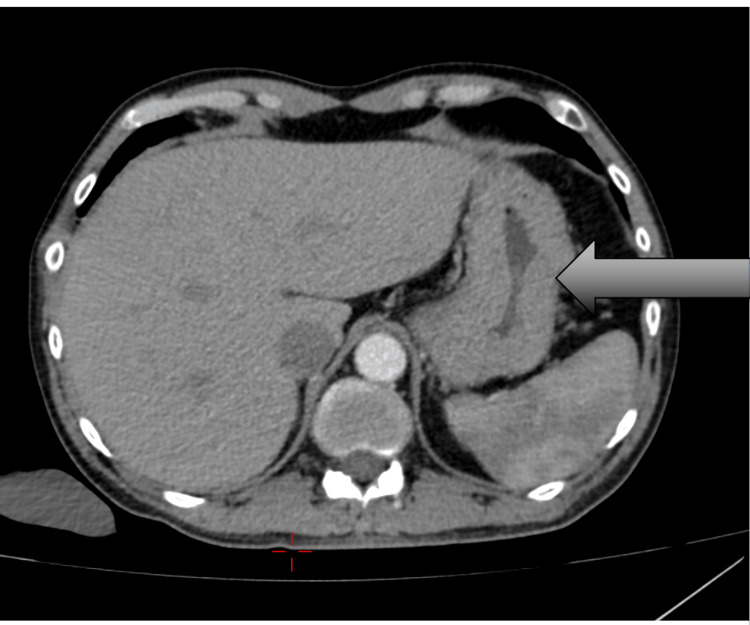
Fundus/body wall thickening seen on CT
